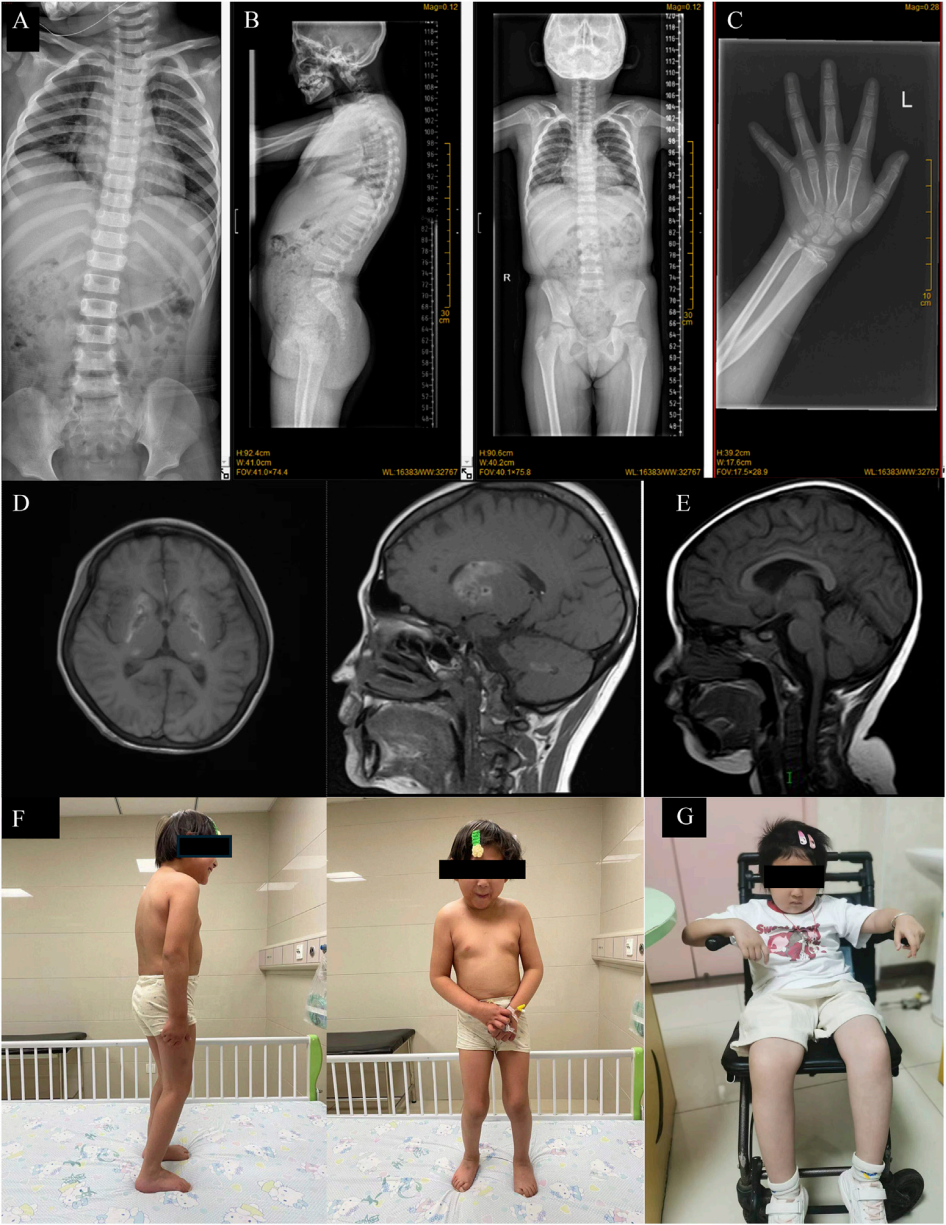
(A) X-Ray of spine of Case2 showing platyspondyly. (B) X-Ray of spine of Case 1 showing platyspondyly. (C) X-Ray of forearm and wrist of Case 1 showing metaphyseal dysplasia. (D) Brain MRI of Case 1 showed the formation of calcification foci in bilateral basal ganglia. (E) Brain MRI of Case 2 showed short T1 signal in bilateral pallidus and high T1WI signal in adenohypophysis. (F) Clinical photos of Case 1, standing relatively reluctantly; (G) Clinical photos of Case 2, in which the patient had been unable to stand on his own since the beginning.
